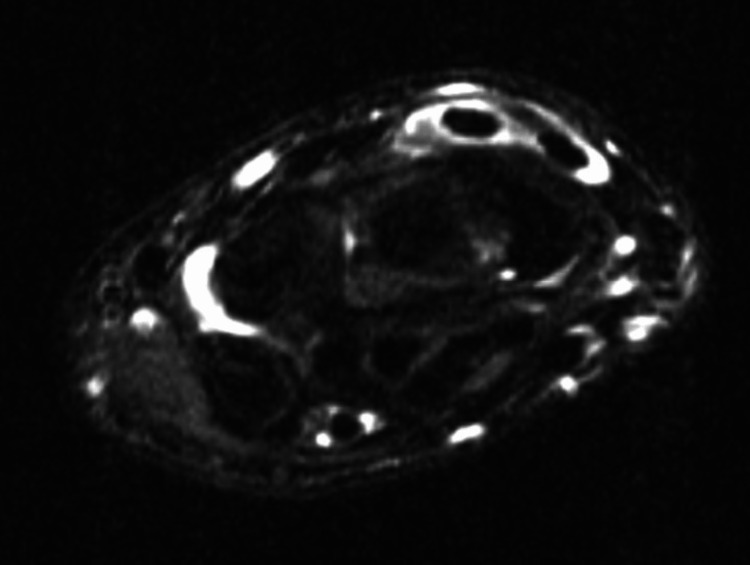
Axial T2-weighted images of the patient’s left demonstrating fluid within the tendon sheaths of the ECRB/ECRL and EPL ECRB: Extensor carpi radialis brevis; ECRL: Extensor carpi radialis longus; EPL: Extensor pollicis longus
